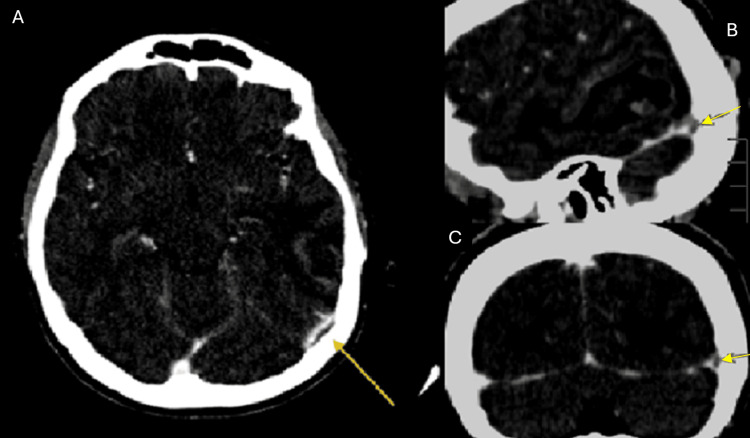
CT venography phase showing (A) axial, (B) sagittal, and (C) coronal views demonstrating a linear flow void in the left transverse sinus (Yellow Arrows).
